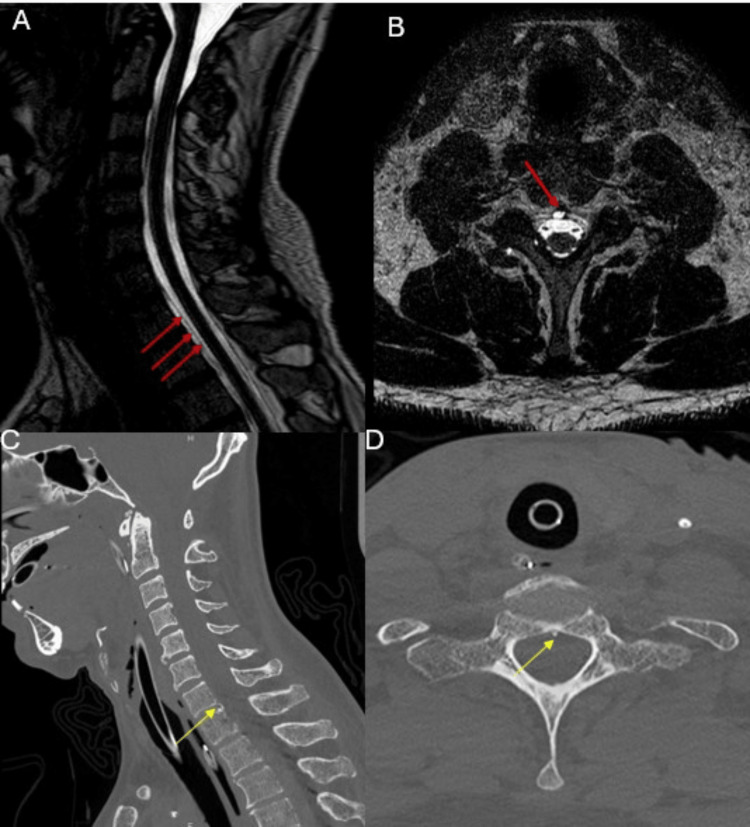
Cervical MRI and CT scan Spinal longitudinal extradural collections (red arrows) are demonstrated on sagittal T2-weighted fast spin-echo (A) and axial T2-weighted (B) MRI. Sagittal (C) and axial (D) cervical spinal CT images reveal a rostrally oriented osteophyte at the C7-T1 level (yellow arrows). MRI: magnetic resonance imaging; CT: computed tomography
